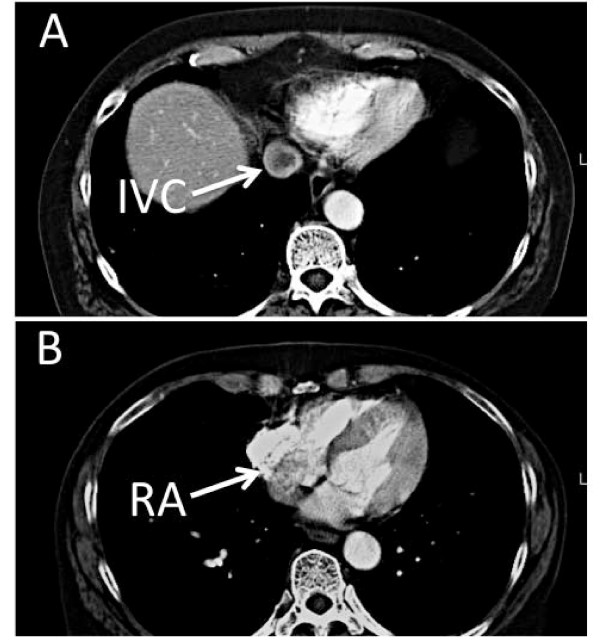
Visualization of the IVC tumor using contrast enhanced axial CT. Panel A: Dynamic contrast-enhanced axial CT scan through the suprahepatic IVC demonstrates central filling defect consistent with tumor or thrombus. Panel B: At an adjacent axial level, CT scan demonstrates a negative filling defect within the right atrium, suggesting the presence of an intra-atrial mass but the image is not conclusive for a cyst in the right atrium especially in comparison with the TEE images. IVC: inferior vena cava, RA: right atrium.
